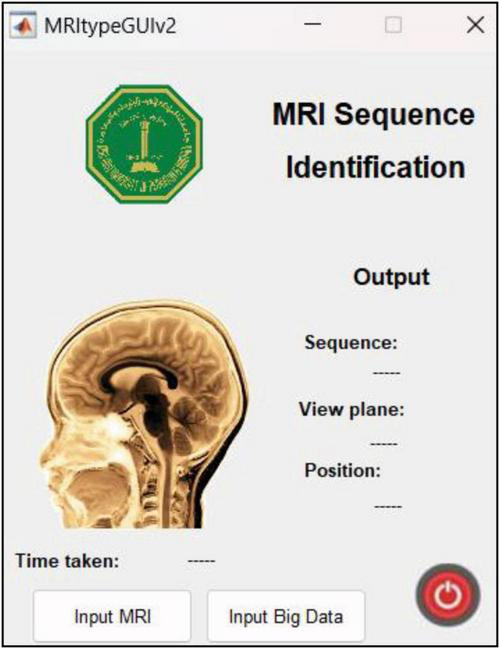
UI designed in MATLAB. The “input MRI” pushbutton accepts MRI file in a dialog box. The “input big data” pushbutton requests for a path to multiple MRI scans and classifies them, thereby reducing time and requiring minimum human intervention. The sequence, view plane and relative slice position are displayed along with the inference time.
